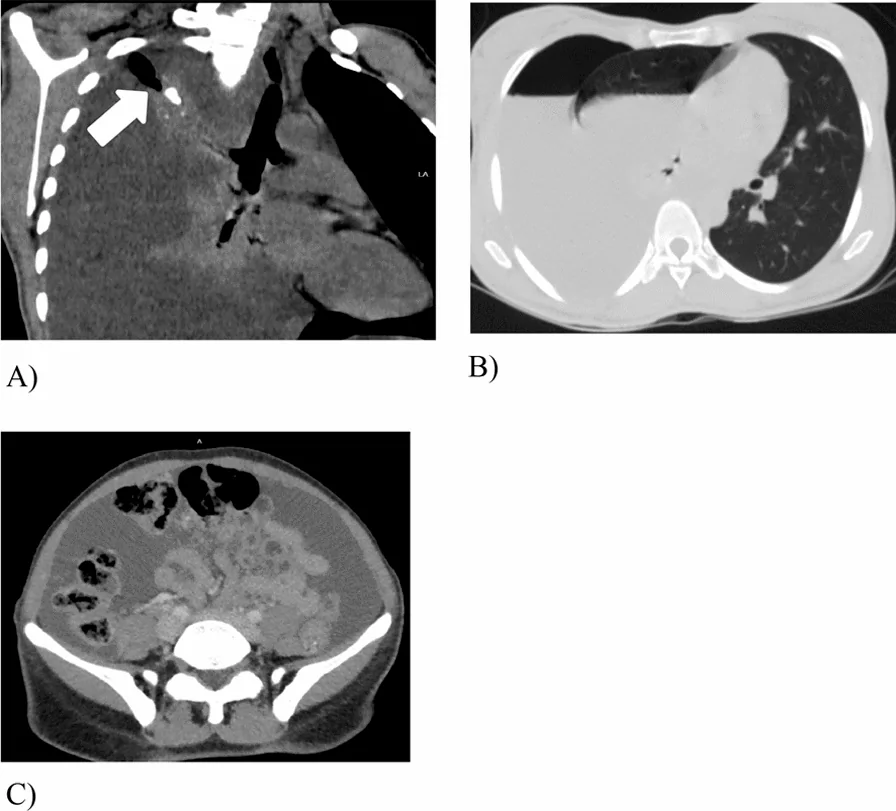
CT scan of the chest and abdomen. A- Chest coronal oblique view images showing broncho-pleural fistula, B- Chest axial view showing marked hydro-pneumothorax and C- Abdomen axial view showing abdominal ascites
